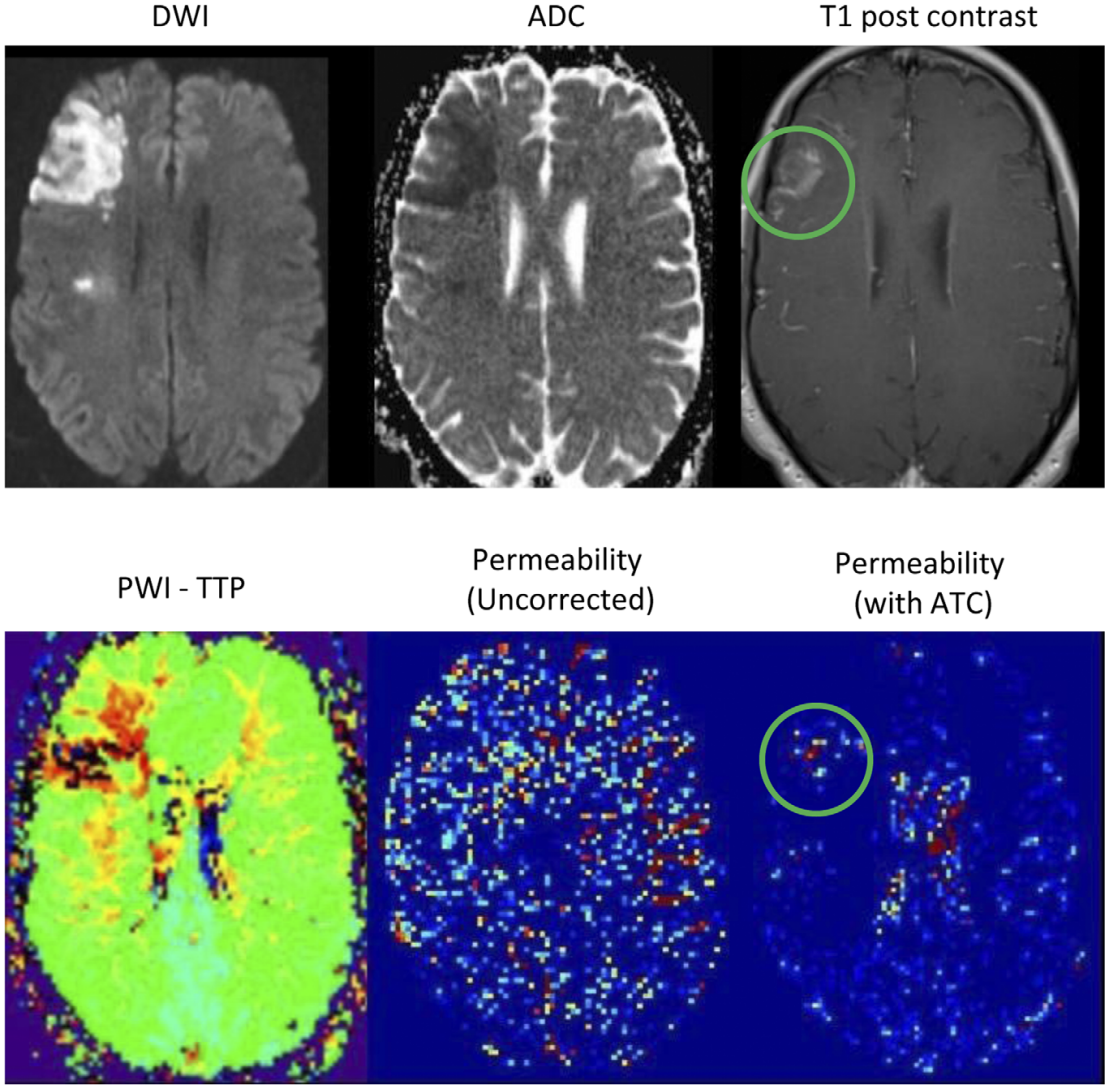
The top row of images show a stroke on DWI/ADC which has some enhancement on post contrast T1 imaging. The bottom row shows the perfusion deficit on a TTP map, the permeability image when not corrected for arrival time, and the permeability image after arrival time correction. The green circles show corresponding areas of contrast leakage on the T1 post contrast and ATC permeability images. (DWI = diffusion weighted image, ADC = apparent diffusion coefficient, PWI = perfusion weighted image, TTP = time to peak, ATC = arrival time corrected).
